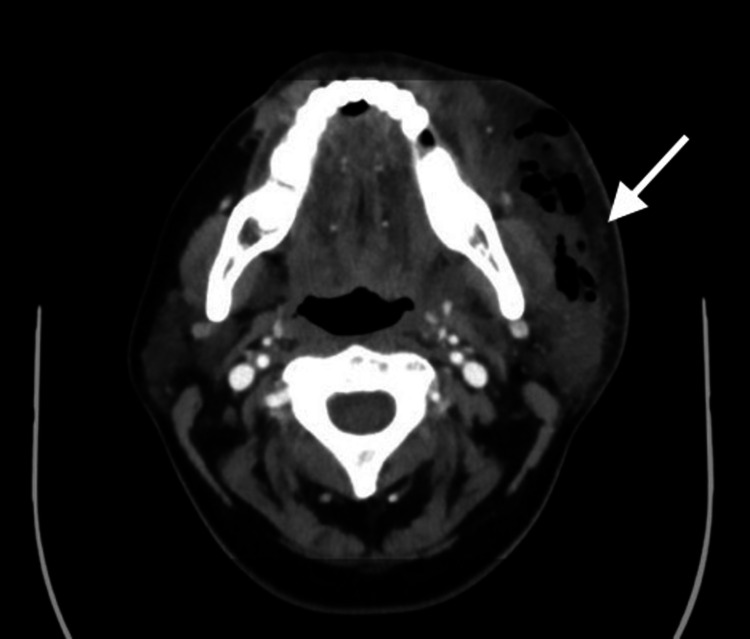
CT scan of the patient's sinuses, facial bones, and neck The scan revealed subcutaneous stranding in numerous pockets of air throughout the left face and neck without fluid collection (arrow) CT: computed tomography
